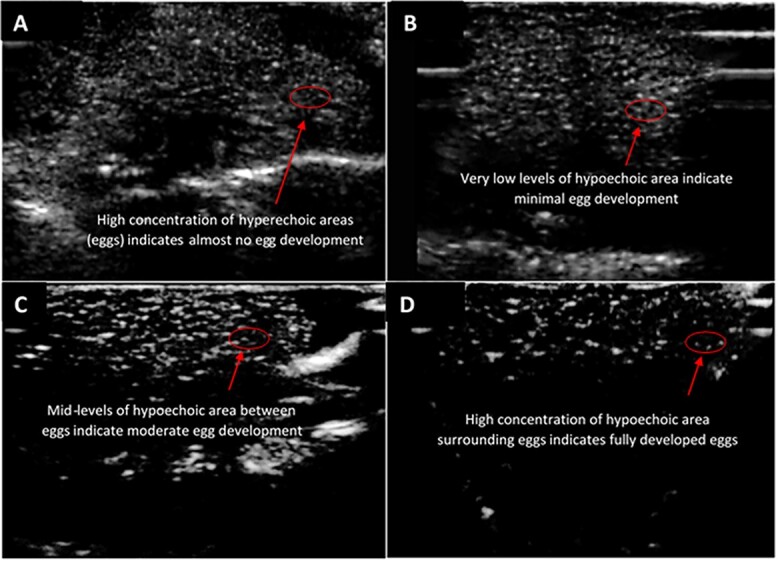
Representative images of grades 0–3 of Fowler’s toads. Egg jelly is indicated by hypoechoic (dark) areas and eggs by hyperechoic (white) areas. (A) Almost no hypoechoic areas between eggs indicate this female has almost no egg development. (B) Low levels of hypoechoic areas between hyperechoic areas indicate minimal egg development. (C) Mid-levels of hypoechoic are between eggs show that this female has moderate egg development and could be administered a priming dose, followed by an ovulatory dose for IVF. (D) High levels of hypoechoic area indicate that this female has fully developed eggs and could be administered an ovulatory dose for IVF. Females at ultrasound grades 2 and 3 were selected for IVF.
